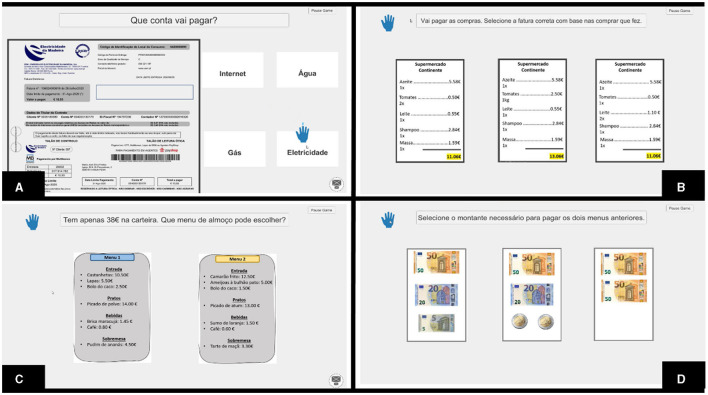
This figure represents four CTTs used in the computerized CT program: (A) Analyze the bill task—participants are required to analyze a specific bill (e.g., internet, electricity, gas) and to answer several questions related to that bill (e.g., What is the total cost of the bill?); (B) Choose the correct invoice—based on the prices of grocery items shown on a from the previous task, participants were required to select the correct invoice among incorrect invoices. To do so, they needed to retain the information regarding the prices and the number of items and then perform some calculations to estimate the total cost of their purchase; (C) Pay the lunch menu(s) task—participants are required to analyze several lunch menus and to select the right menu(s) considering the amount of money available; and (D) Pay the lunch—participants must select the correct amount of money needed to pay the previously selected lunch menu(s).
